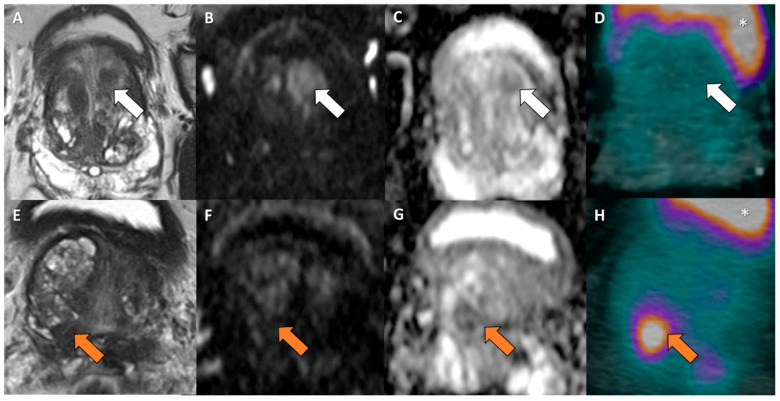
Comparison of two examples with extreme pathology results: (A–D) mpMRI-positive (PI-RADS 5) and PSMA-negative (PRIMARY score 1) of a patient with negative biopsy; (E–H) mpMRI-negative (PI-RADS 3) and PSMA-positive (PRIMARY score 5) of a patient with ISUP 5 (GS 9) adenocarcinoma of the prostate; (A,E) T2-weighted image with prostate; (B,F) diffusion-weighted imaging (DWI); (C,G) apparent diffusion coefficient (ADC); (D,H) axial fused [^68^Ga]PSMA-11 PET/CT (* defines the uptake in the bladder; arrows highlight the target lesions). Abbreviations: mpMRI = multiparametric magnetic resonance imaging; PI-RADS = Prostate Imaging–Reporting and Data System; ISUP = International Society of Urological Pathology; GS = Gleasons score.
